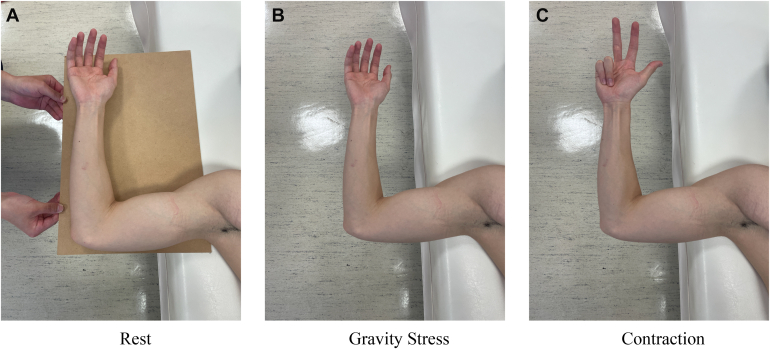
Measurement of medial joint space distance of the elbow under different conditions. Ultrasonographic evaluation of the medial joint space distance under 3 conditions: (A) at rest (gravity eliminated), (B) with gravity stress, and (C) during contraction of the forearm flexor–pronator muscles (performed by flexing the fourth and fifth proximal interphalangeal joints).
